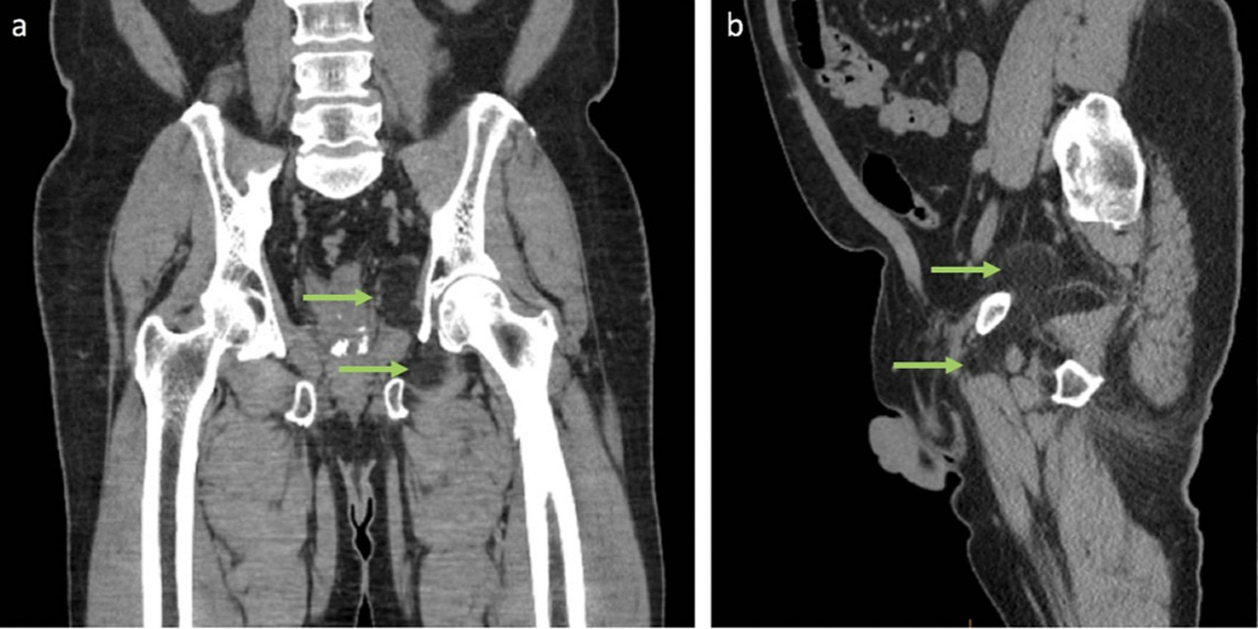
(a – b) Computed tomography (CT) images of the pelvis with coronal and sagittal views showing a bilobed, dumbbell-shaped fat density lesion in the left obturator canal (green arrows).
